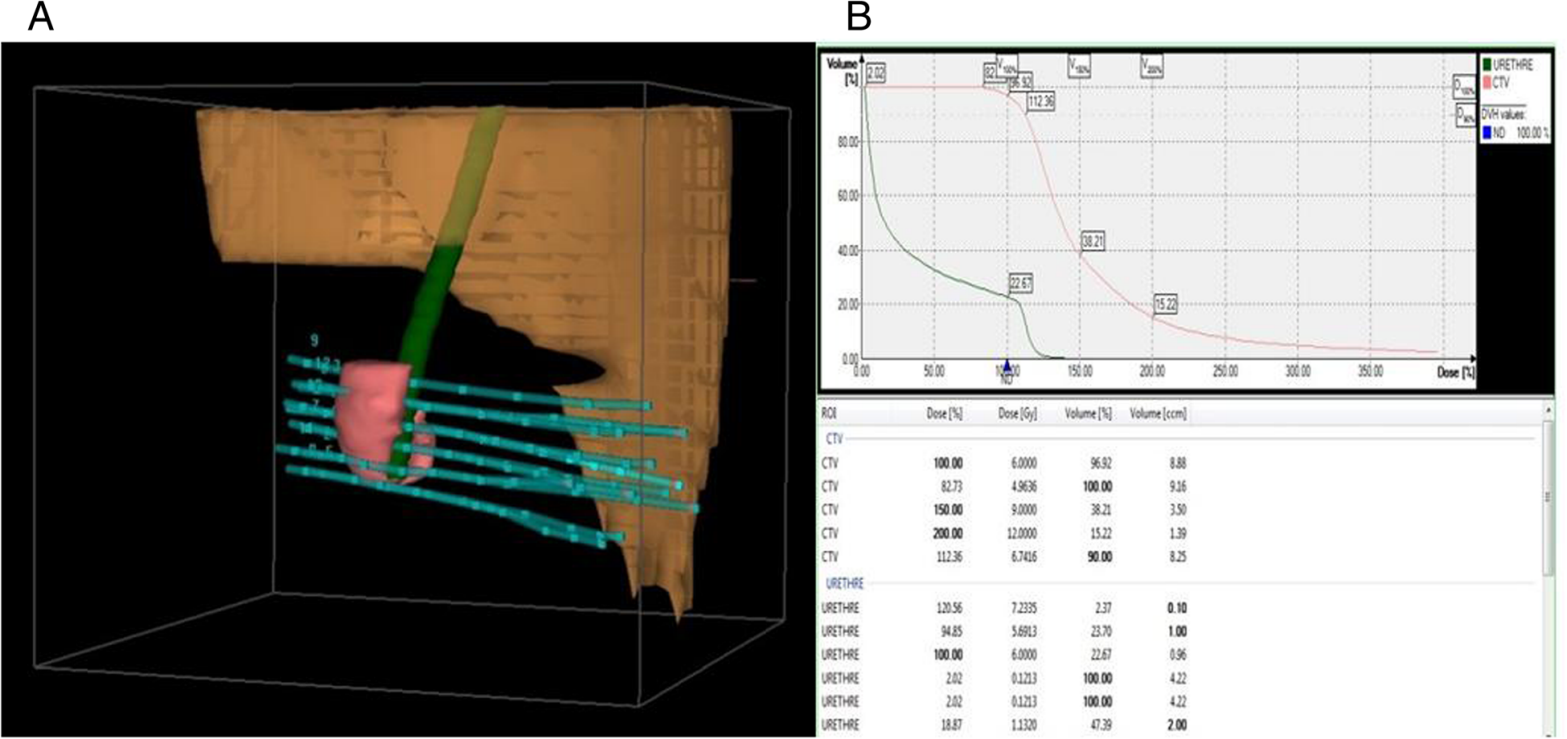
Post-implant CT-scan 3D reconstruction (A), dose-volume histogram (B).
